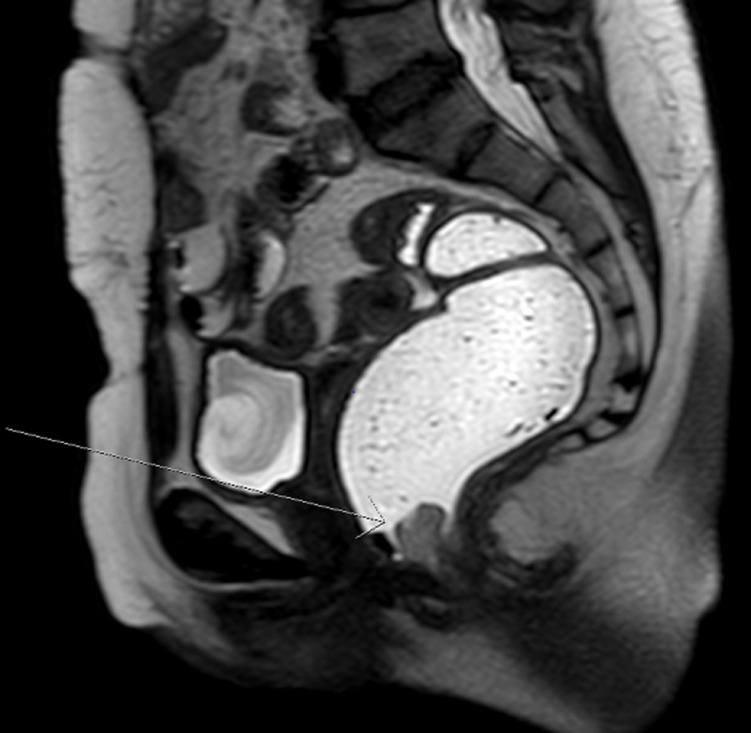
MRI pelvis, sagittal T2 weighted image showing lobulated enhancing mass arising from the lower rectum close to the anal sphincter
